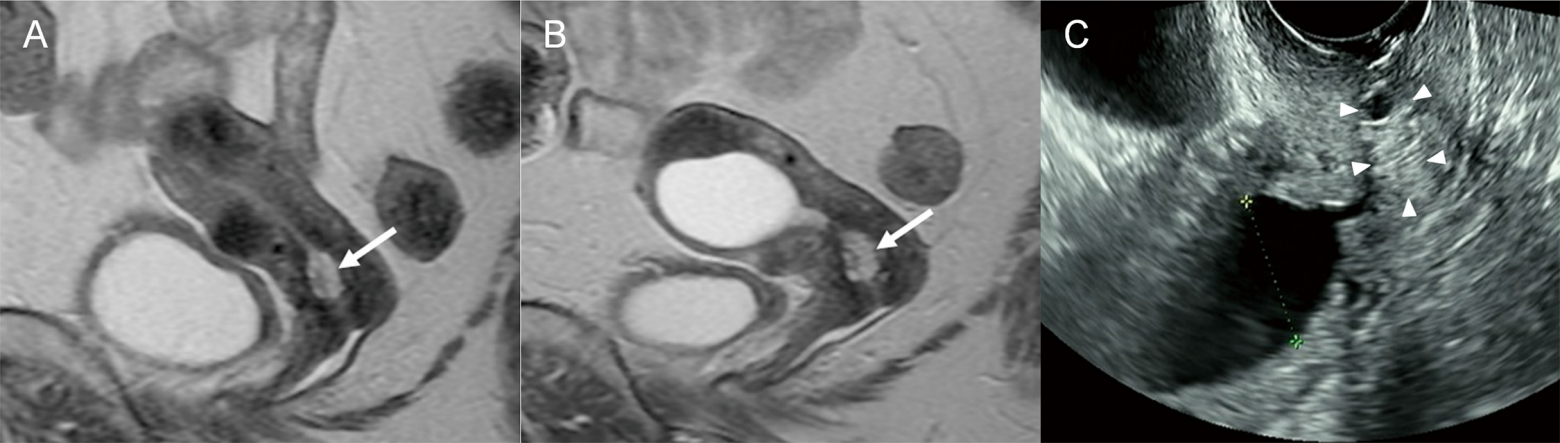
Raspberry-type multicystic lesion of the cervix. (Case 18) A. Magnetic resonance (MR) image at the initial visit shows a lesion of numerous, closely aggregated tiny cysts (arrow) with hyperintensity on T2-weighted images. B. MR image before hysterectomy shows hydrometra in addition to a raspberry-type multicysytic lesion (arrow) with little change in size and shape. C. Transvaginal sonography shows hydrometra and a hyperechoic lesion that includes a small cyst (arrow heads) in the endocervix.
